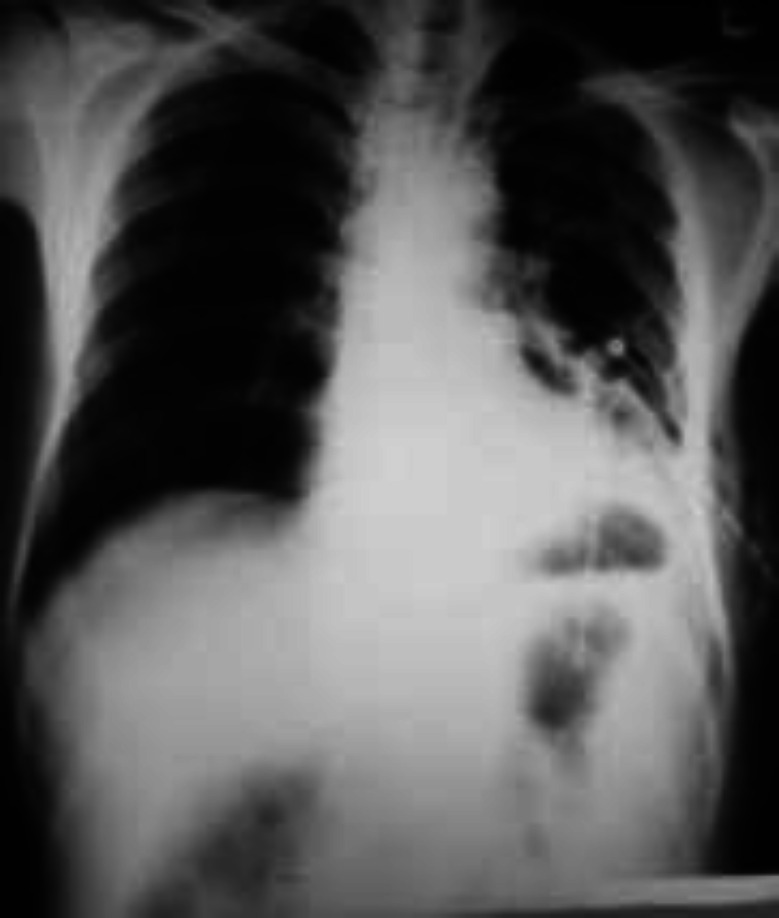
Chest radiograph after laparatomy revealing expanded left lung with chest tube in the posterior recess of the diaphragm
